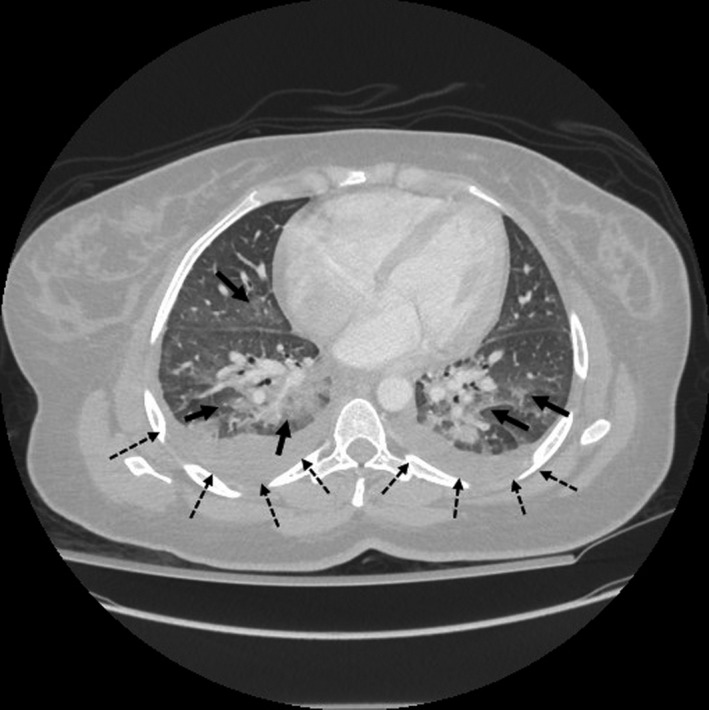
Computed tomography scan of the chest of a 37‐year‐old woman with Lemierre's syndrome shows multifocal pneumonia (solid arrows) with bilateral pleural effusion (dashed arrows).
